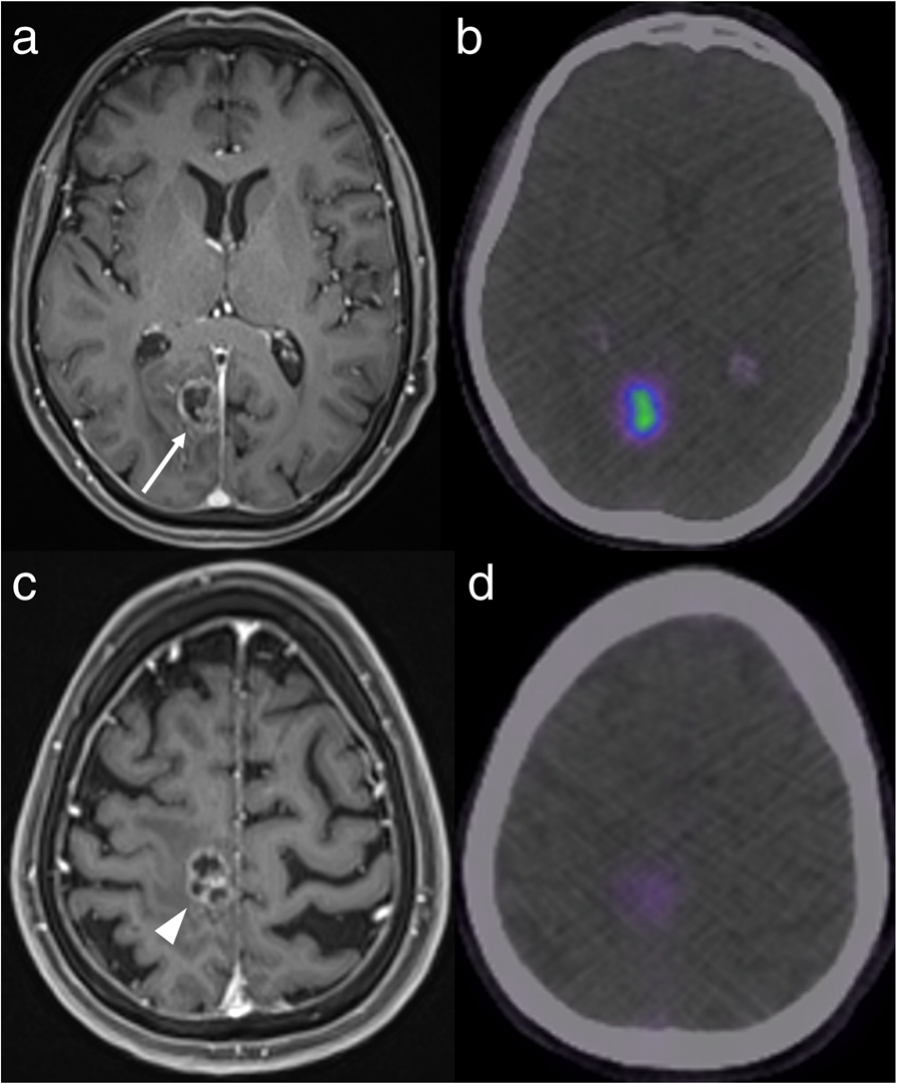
Corresponding post-contrast MRI (left) and GaTate-PET (right) images of two cerebral metastases in a patient with metastatic NET. The medial right occipital metastasis (a; arrow) demonstrates high GaTate uptake (b). If this were a solitary metastasis, a response to PRRT would be expected. The medial right pre-central gyrus metastasis (c; arrowhead), however, demonstrates low GaTate uptake (d), and is unlikely to respond to PRRT
